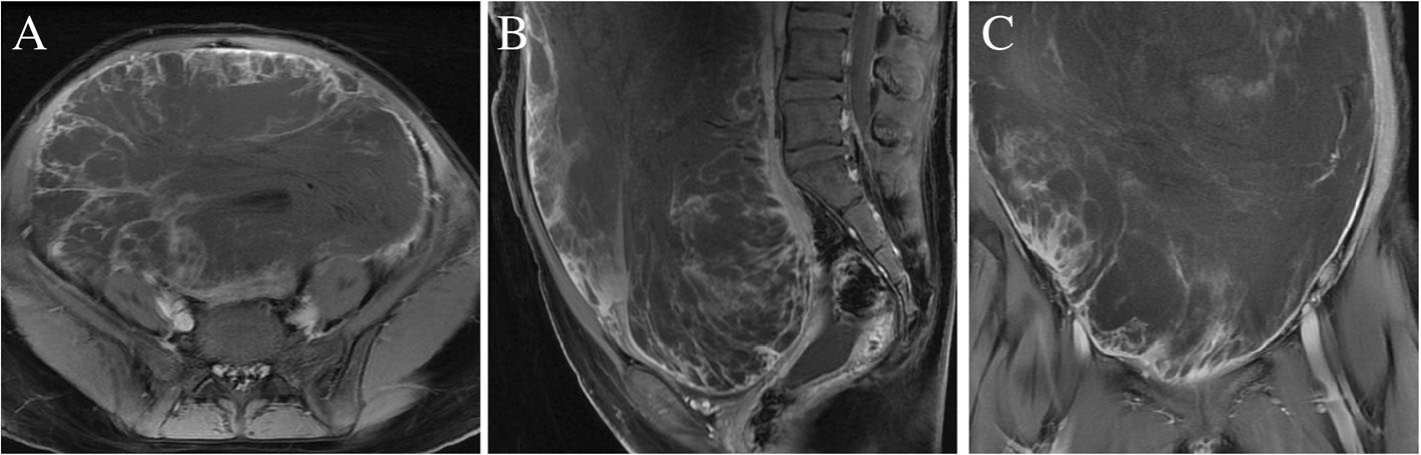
T2 Magnetic resonance imaging with enhancement of the pelvic mass in case 4 (description refers to the main text). a Axial view. b Sagittal view. c Coronal view
